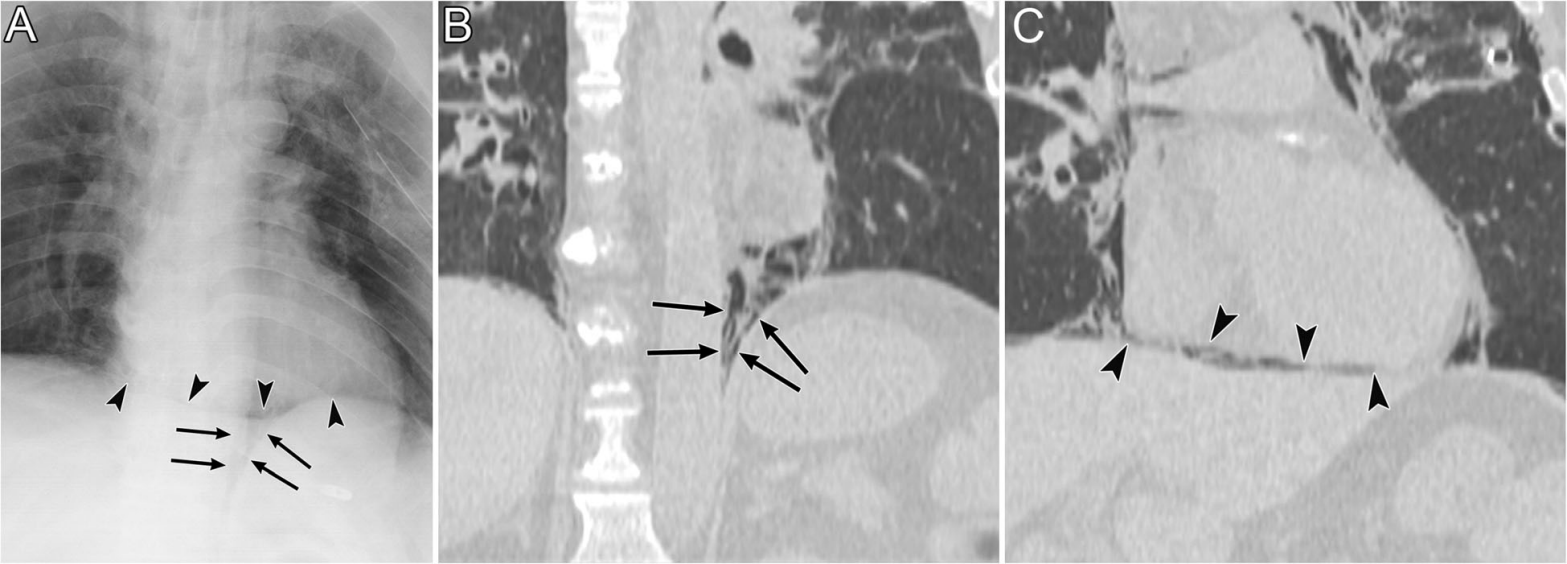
Pneumomediastinum in a 50-year-old man. The pneumomediastinum was caused by tracheostomy displacement. a A supine radiograph shows pneumomediastinum and subcutaneous emphysema. Air along the left aortic border and the medial left hemidiaphragm become V-shaped (i.e., “Naclerio’s V” sign) (arrows). The “continuous diaphragm” sign (arrowheads) is also visible. b A coronal CT image of the lung base reveals “Naclerio’s V” sign (arrows). c Another coronal CT image of the lung base reveals a “continuous diaphragm” sign (arrowheads)
